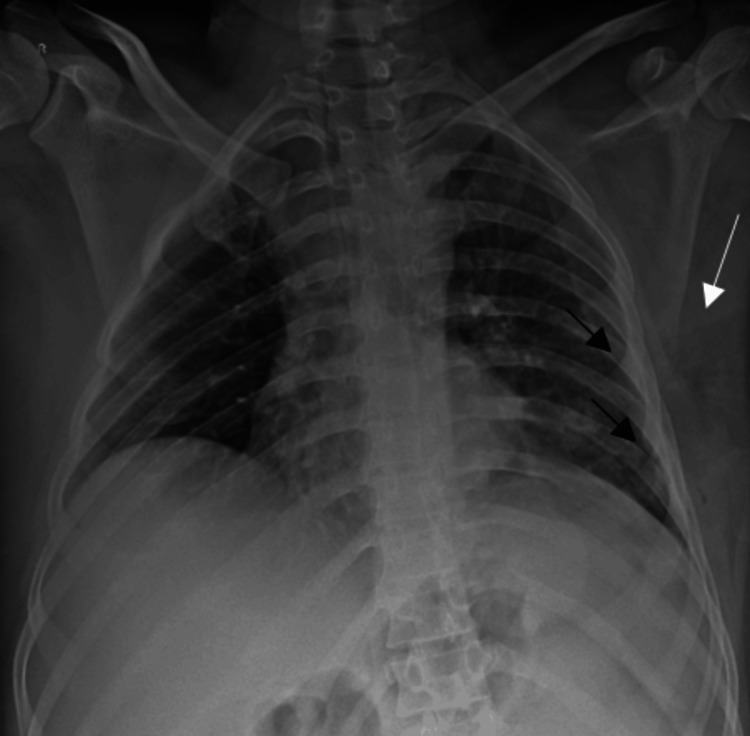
Chest radiograph taken in the supine position shows a few inhomogeneous opacities in the left middle and lower zones with a suspicious ill-defined lucency in the subcutaneous tissues of the left chest wall (white arrow). Linear displaced fractures of the left fourth and fifth ribs are also seen (black arrow).
